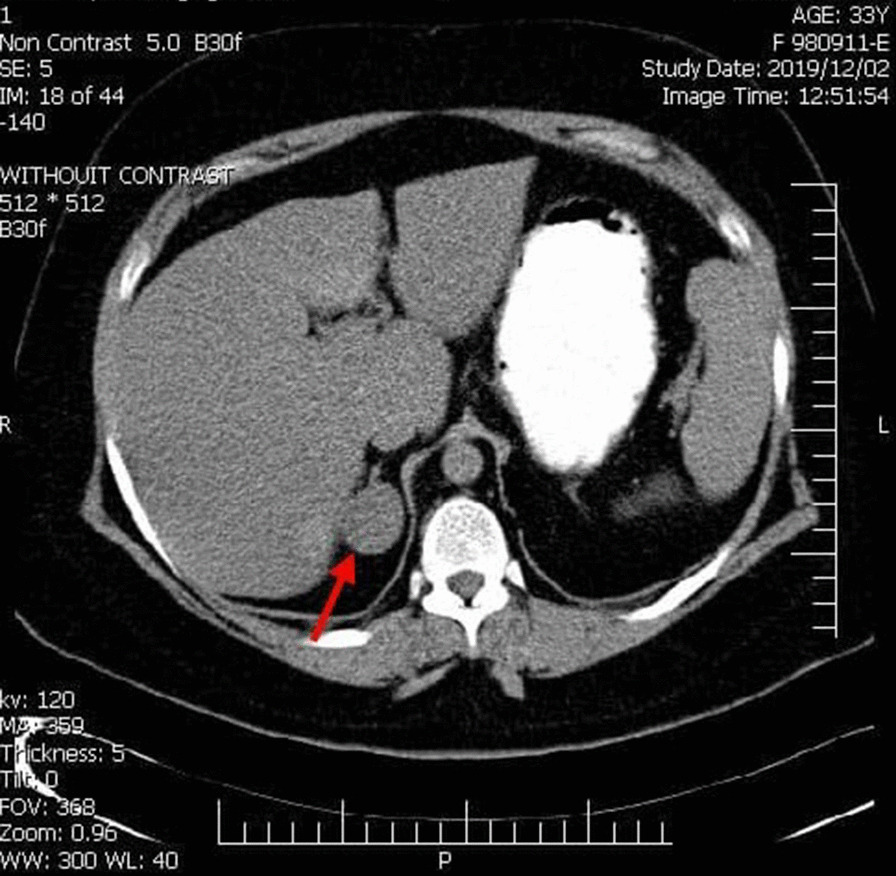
Spiral computed tomography of the abdomen using injection contrast: arrow shows a 3.5 cm diameter mass with a regular margin and significant hemogenic density in the right adrenal gland
